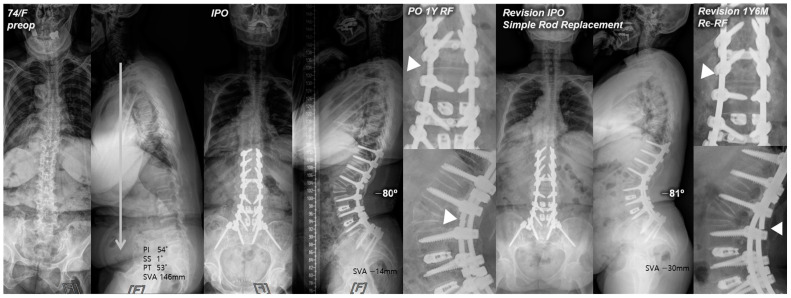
Pre- and postoperative standing radiographs of a 74-year-old female patient. After T10-S1 posterior instrumentation with PSO on L2, PLIF on L3–5, and ALIF on L5-S1, optimal sagittal balance was achieved (SVA, −14 mm; TK, 28°; LL, −80°; PI, 54°; PT, 4°; SS, 50°). At 1 year after primary deformity correction, RF (left rod) occurred at L2. At 1 year and 6 months following revision surgery with simple bilateral rod replacement, re-RF occurred at L2–3. White triangles indicate the site of RF.
